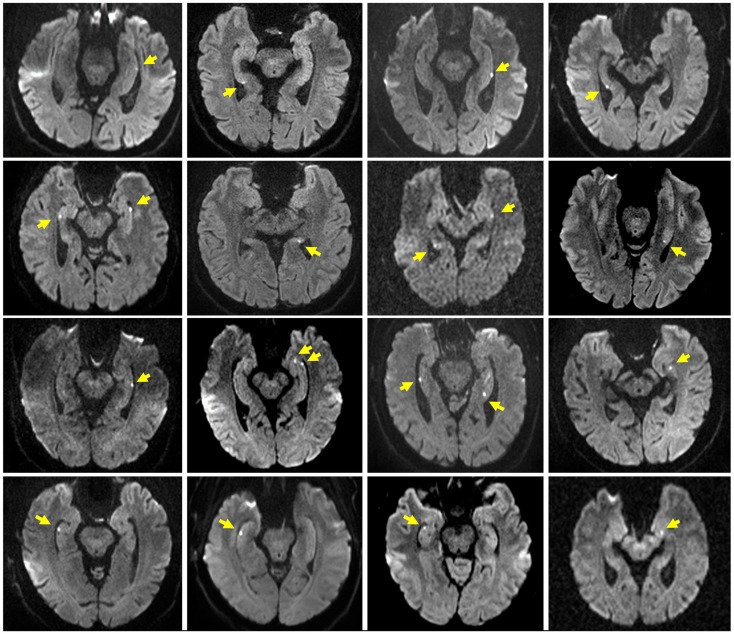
Hippocampal MRI in TGA patients. Representative diffusion-weighted MR images after the acute episode of TGA in 16 of the 20 patients. Slice positioning parallel to the long axis of the hippocampus. Yellow arrows indicate the hippocampal lesions.
